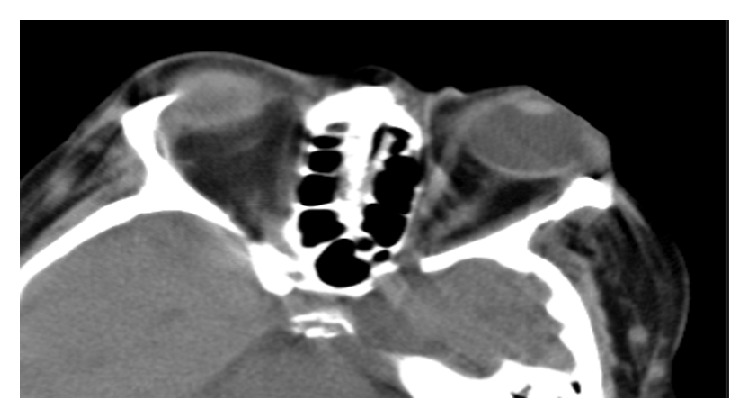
CT scan of the orbits showing bilateral preseptal soft tissue swelling, more on the left side, soft tissue stranding in left intra- and extraconal fat, and left-sided mild proptosis.
